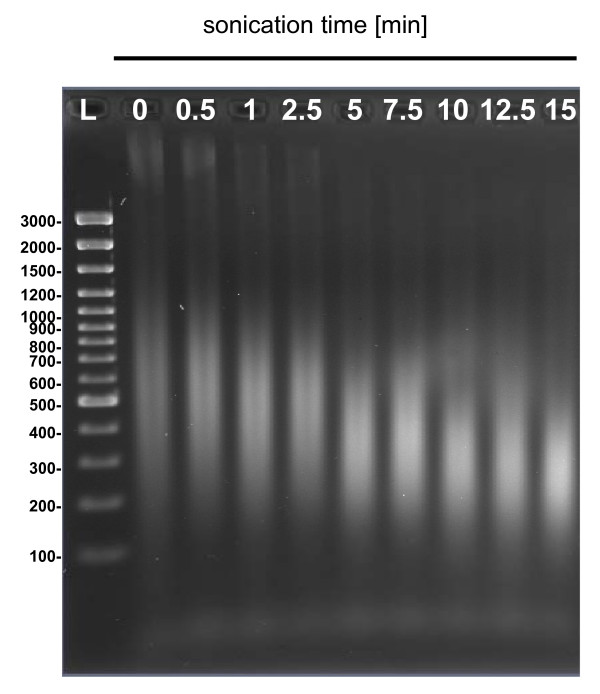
Electrophoretic analyses of distribution of genomic DNA of *E. coli *EDL933 subjected to 0 – 15 min ultrasonication. L indicates the DNA Ladder.
